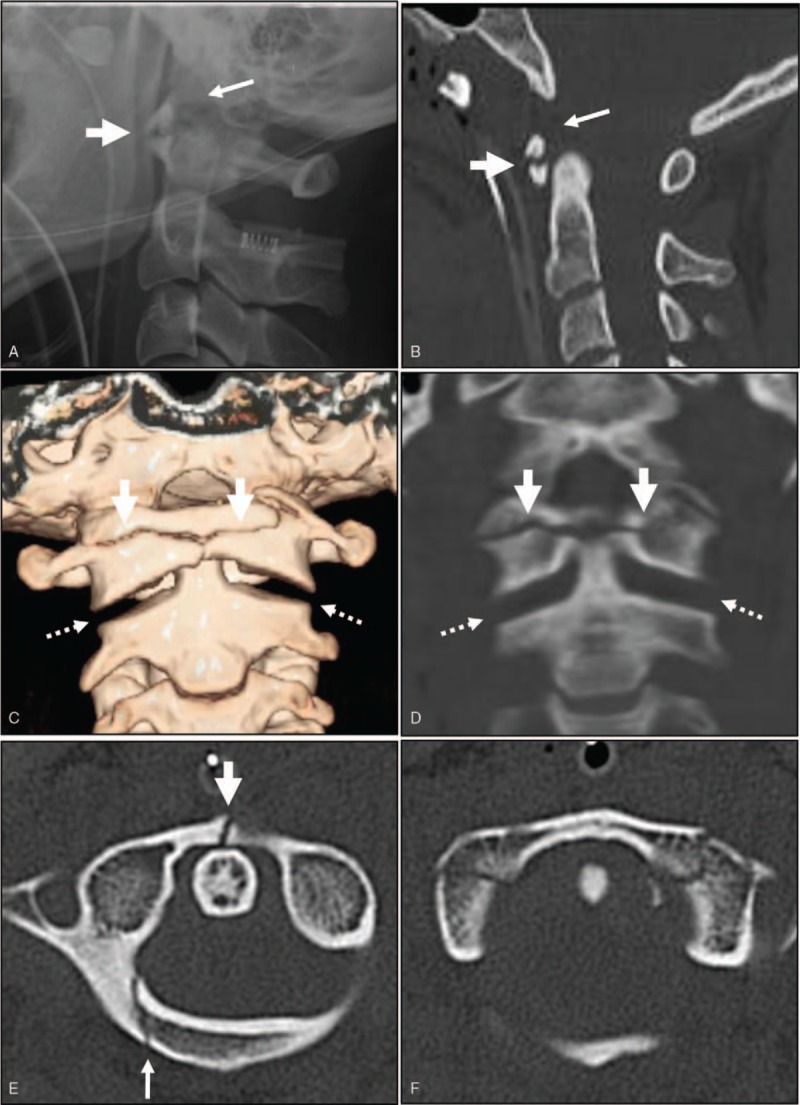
Lateral radiograph and sagittal 2-D computed tomography (CT) revealed horizontal split fracture and upward displacement of the anterior arch of the atlas (thick arrow), as well as an increased basion-dental interval of 18 mm (arrow) (A and B). Coronal 3-D and 2-D CT revealed horizontal split fracture of the anterior arch of the atlas (thick arrow) and increased atlanto-axial joints (dotted arrows) (C and D). Axial CT revealed 2-part fracture of the atlas including anterior arch fracture (thick arrow) and posterior arch fracture (arrow) (E and F).
